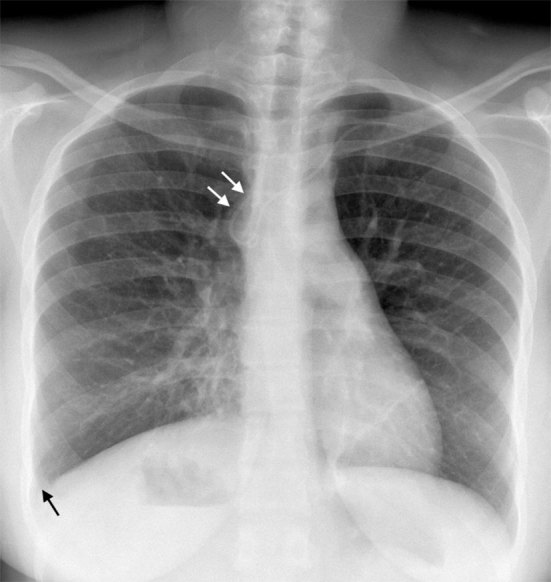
Frontal chest radiograph 9 days after peripherally inserted central venous catheter insertion shows a small right-sided pleural effusion (black arrow) and a curve in the distal catheter tip (white arrows). The heart is normally oriented but the gastric bubble is under the right hemidiaphragm, consistent with situs ambiguus.
